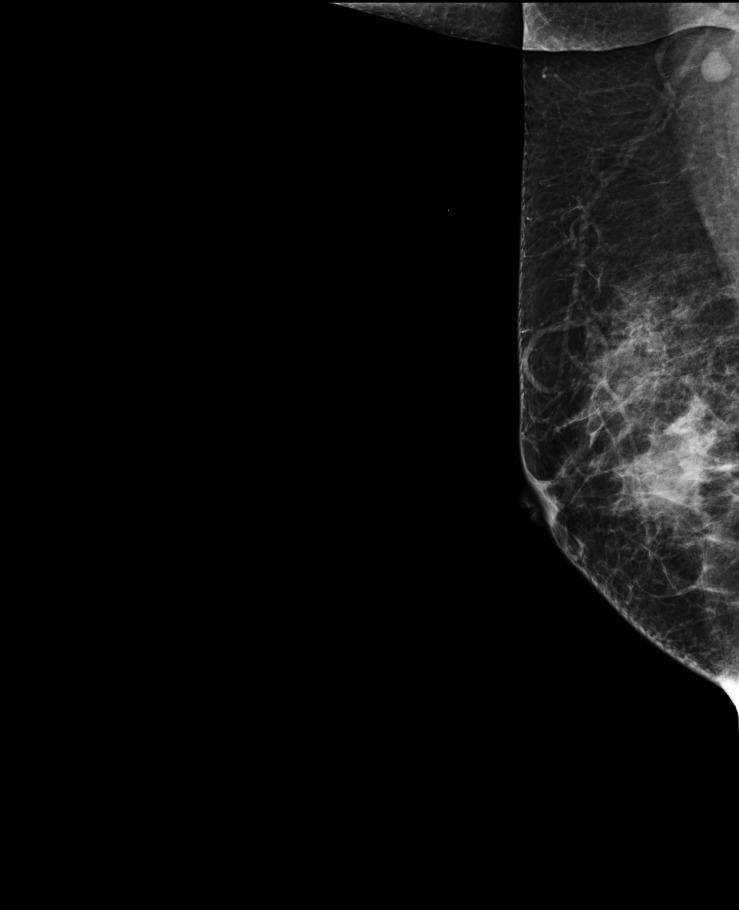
X-ray image of right breast mass and axillary lymph nodes: The structure of the local glands in the upper quadrant of the right breast was slightly disordered with increased density; lymph node shadows were seen in the right axilla with increased density.
